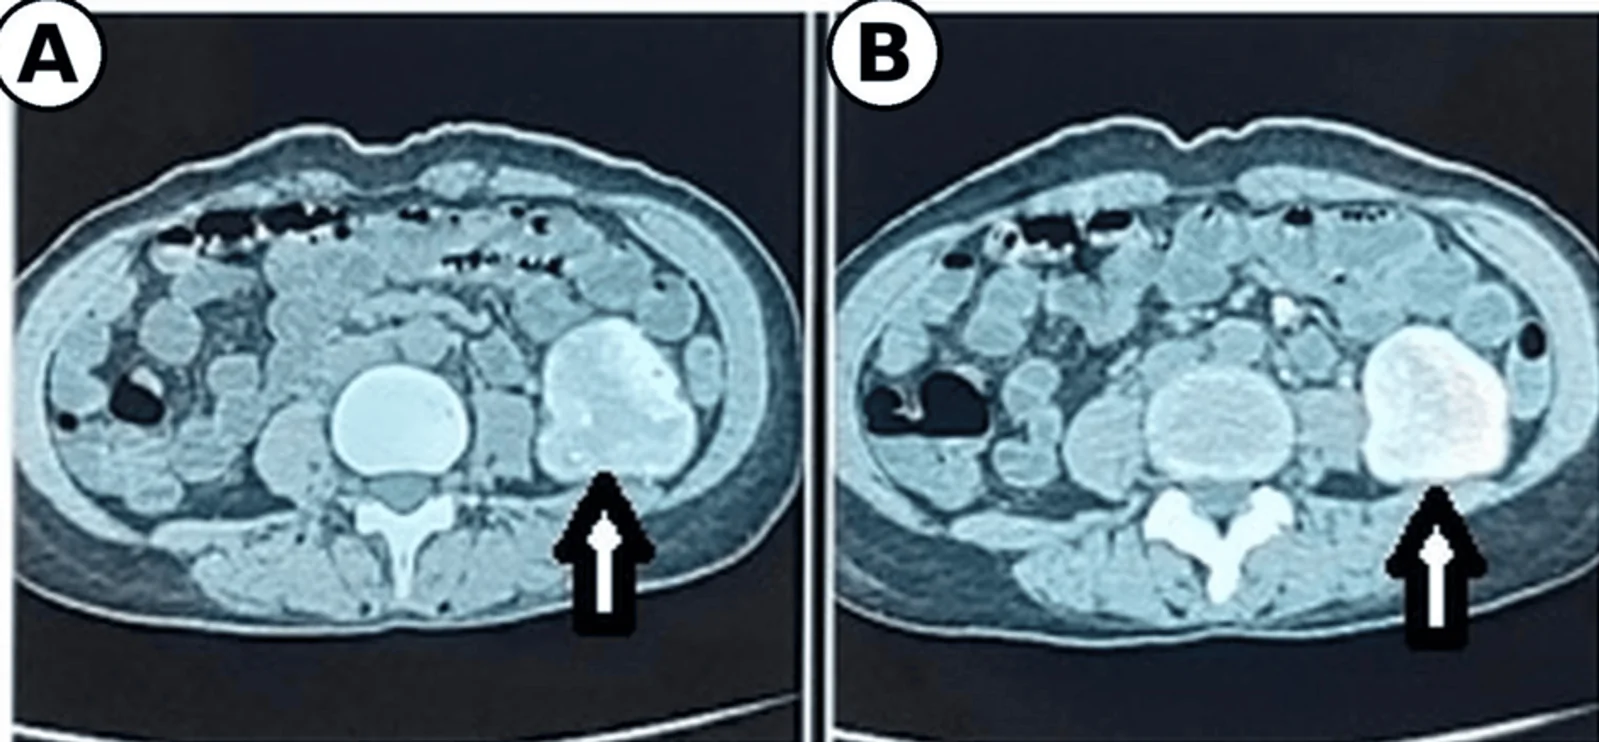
Contrast-enhanced CT of abdomen and pelvis showing a calcified left kidney (solid white arrows) Axial contrast-enhanced CT image (A) showing a calcified left kidney and (B) at an adjacent level, again showing the calcified left kidney with associated arrow marking CT: computed tomography
